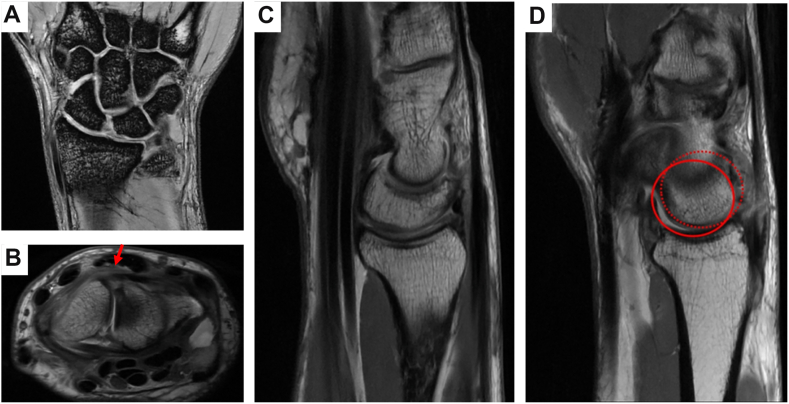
Magnetic resonance imaging of wrist with scapholunate interosseous ligament (SLIL) injury. A Coronal, B axial, and C, D sagittal imaging demonstrating disruption of the SLIL. B Disruption of the SLIL denoted by the red arrow. C Dorsal tilt of the lunate suggests dorsal intercalated segment instability. D Dorsal scaphoid translation.
